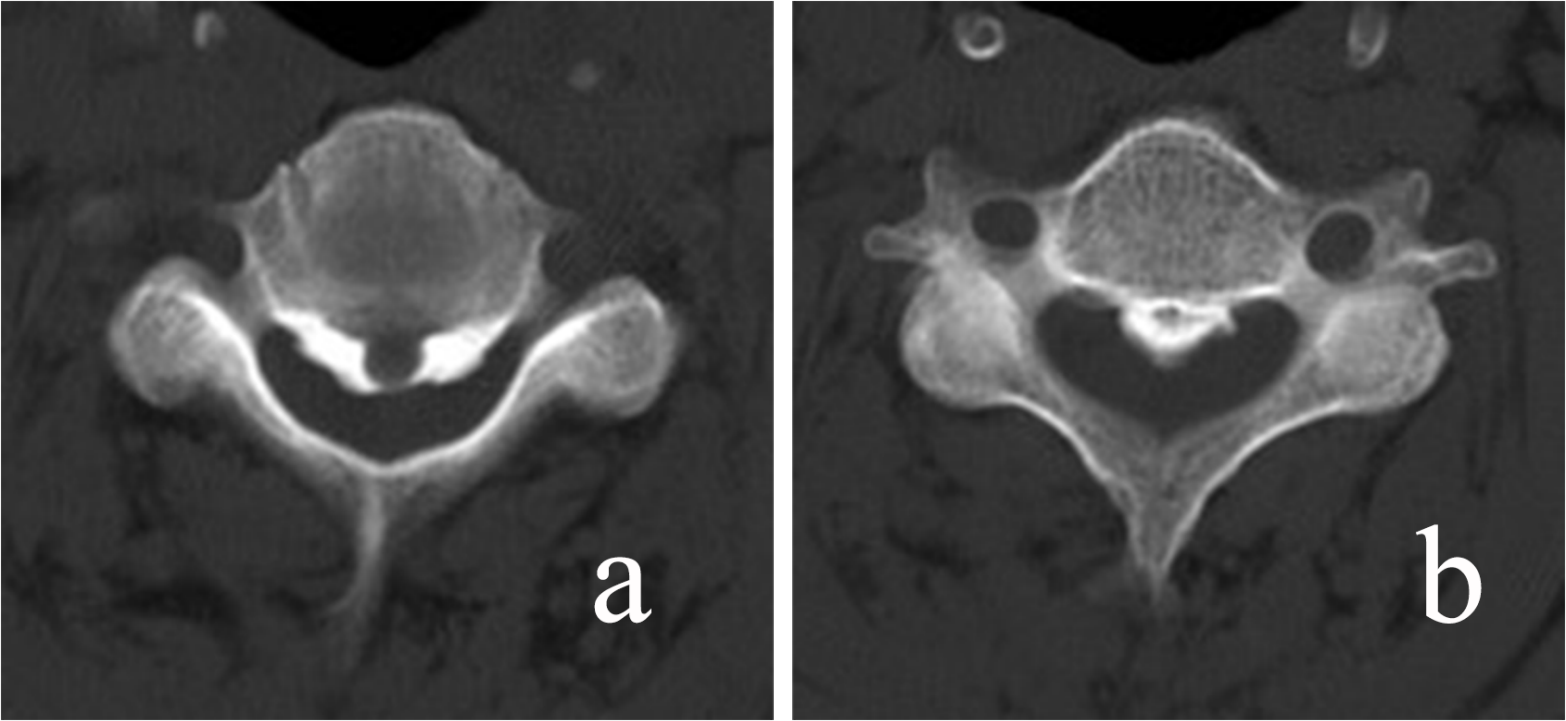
The shape of ossification was defined as the wide-base type and narrow-base type on CT axial imaging.
